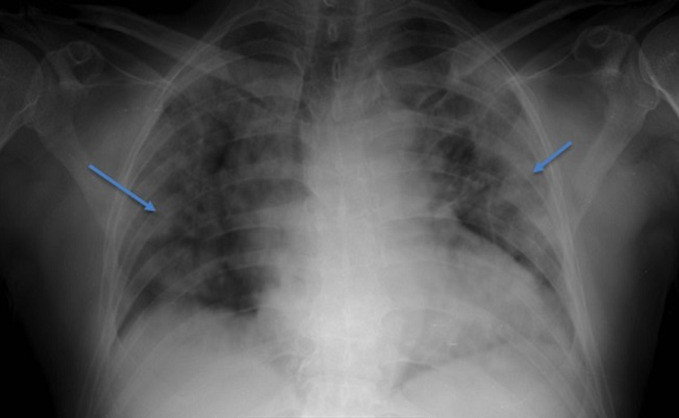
X-ray Chest showing bilateral patchy infiltrates, cardiomegaly, and unfolded aorta are seen
